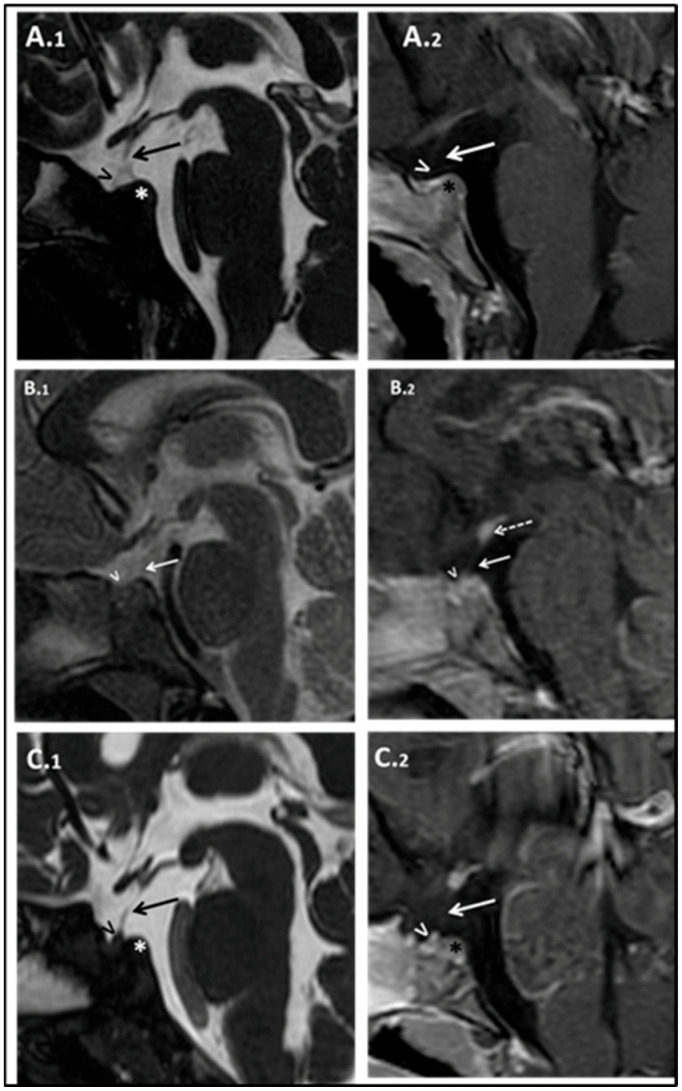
Patient’s brain MRI studies. A.III.1’s Sagittal MRI images T2 weighted (A.1) and T1 weighted with gadolinium (A.2). Subject B.III.2. Sagittal MRI images T2 weighted (B.1), and T1 weighted with gadolinium (B.2). B.III.3 Brain MRI. Sagittal MRI images T2 weighted (C.1) and T1 weighted with gadolinium (C.2). Hypoplastic anterior pituitary lobe (indicated by “>”), small poorly developed sella turcica (indicated by “*”), absent pituitary stalk (see the “arrow”), and ectopic posterior pituitary located at the level of the median eminence.
